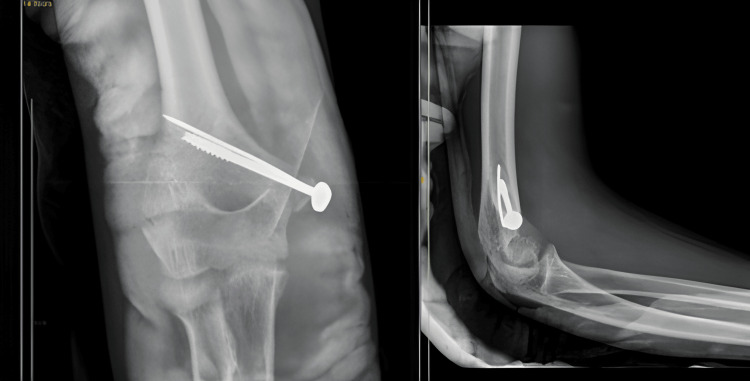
Postoperative X-ray of case 4
